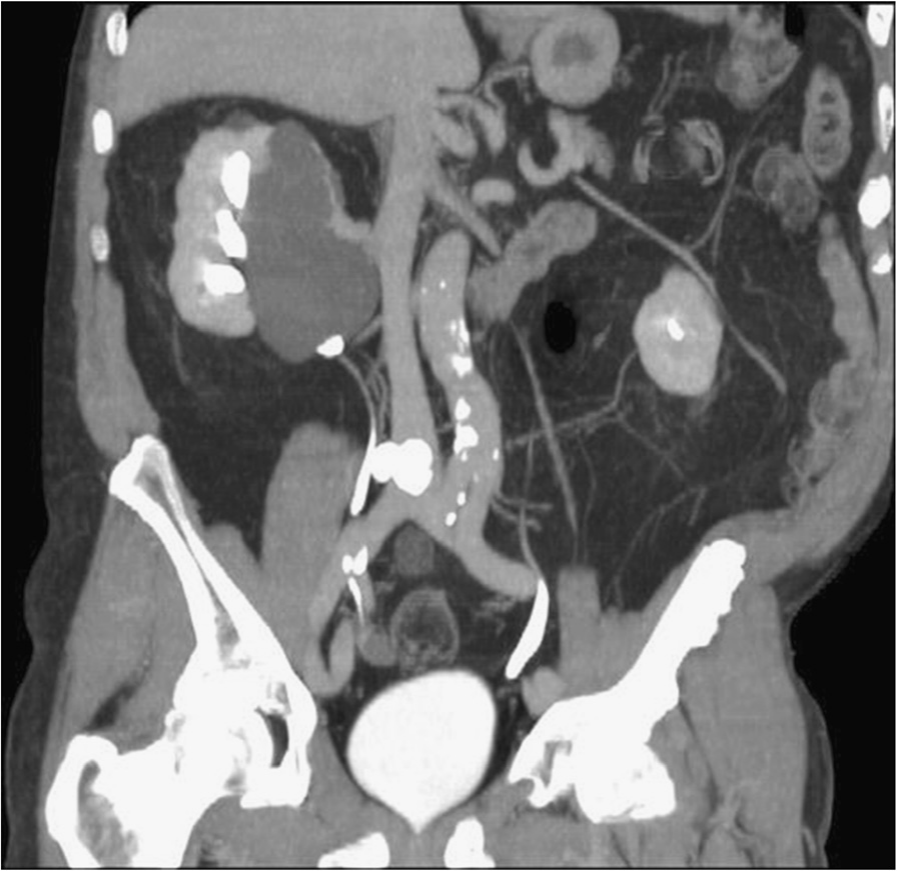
Four months older computed tomography scan (coronal maximum intension projection reconstruction of the excretory phase). This previous computed tomography scan demonstrates a large non-complicated pelvicalyceal cyst on the right kidney with no signs of communication with the collector system since it is not filled with contrast media.
